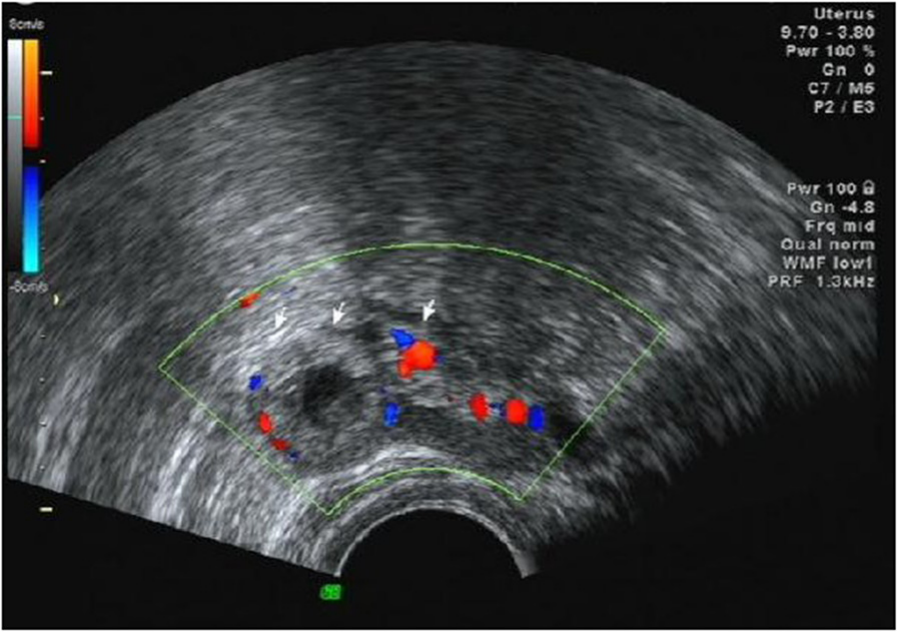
An ultrasound scan (on 18 December 2013) showed a 26mm×16mm left adnexal mass.
